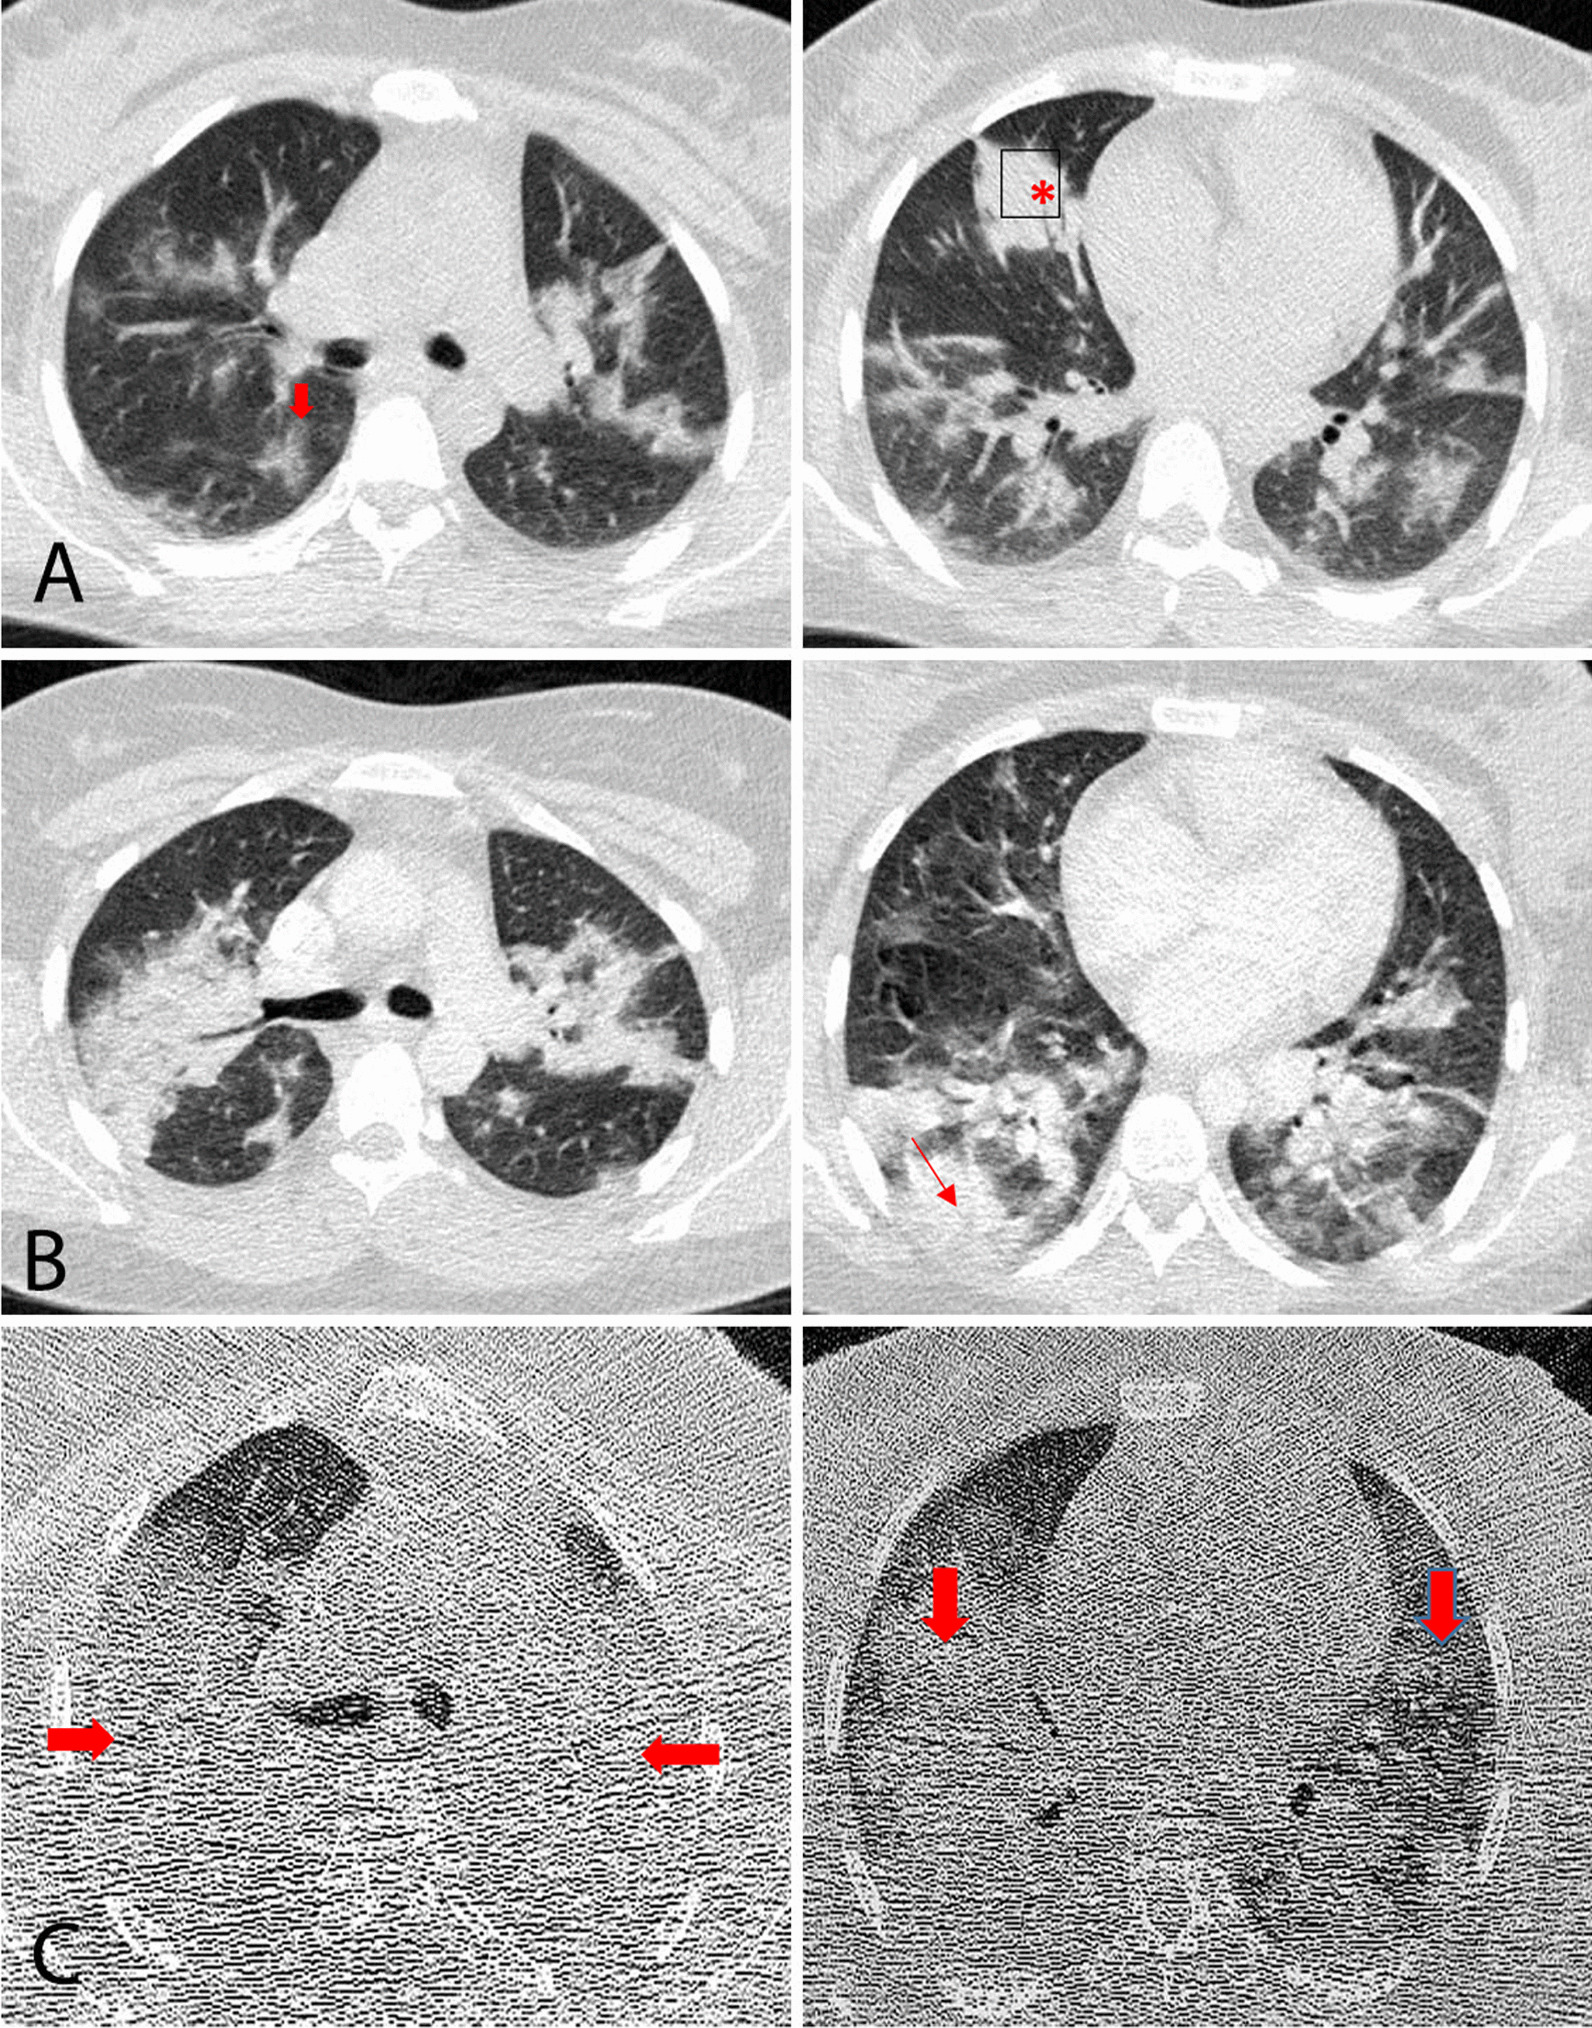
A Axial CT image from day 1 of hospitalization showing multifocal ground-glass opacities (
